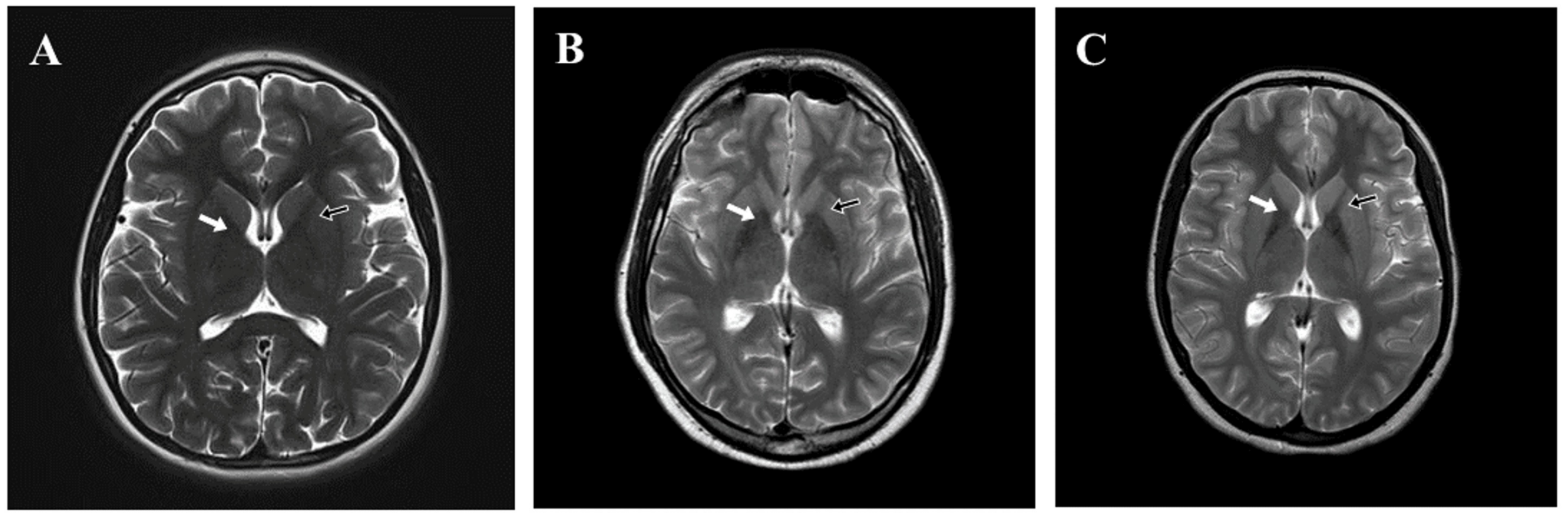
Normal T2-weighted axial brain MRI of patients with Wilson disease. MRI of (A) Patient 4, (B) Patient 5, and (C) Patient 8 showing normal globus pallidus (white arrows) and putamen (black arrows). MRI: magnetic resonance imaging
